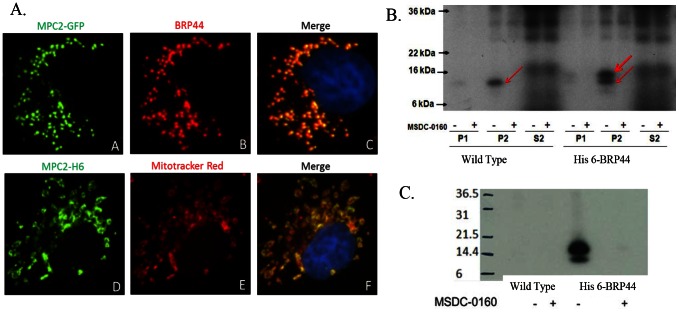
Expressed BRP44 goes to mitochondrion and is crosslinked selectively by the TZD probe. (A) Upper frame: C-terminally-tagged GFP BRP44 (green) in Cos7 cells is co-localized with endogenous BRP44 (red). Lower frame: C-terminally tagged 6×His BRP44 (green) is co-localized with Mitotracker Red confirming the mitochondrial localization. (B) A sequence encoding BRP44 C-terminally tagged with six histidine residues was transiently expressed in HEK293 cells. Subcellular fractions were prepared (P1, crude nuclear fraction; P2, crude mitochondrial fraction; S2, post mitochondrial fraction) and incubated with the radiolabeled photo-probe in the presence or absence of 25 µM MSDC-0160 as indicated. Samples were subjected to one dimensional SDS-PAGE and the dried gel was exposed to X-ray film. The red arrows point to the image of the selectively crosslinked native and C-terminal extension proteins. (C) Crosslinked BRP44 6×His is selectively immunoprecipitated by antibodies to 6×His. Membranes from HEK293 cells stably transfected with BRP44 6×His (right lanes, 6 His) or non transfected cells (left lanes, control) were crosslinked with or without competition with MSDC-0160 as in B. The membranes, all of which contained the specifically crosslinked band as shown in B, were then solubilized and immunoprecipitation was carried out with anti-His agarose beads. The resulting pellets were solubilized and submitted to SDS-PAGE. The corresponding autoradiogram is shown demonstrating that the radioactive band is only precipitated by anti-His tag in the tissue from the cells expressing His-6 BRP44.
